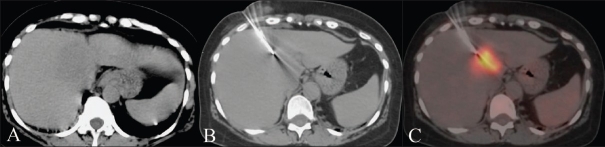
Noncontrast CT scan of the liver (A) during a percutaneous biopsy showing an area of subtle hypodensity which is nearly indistinct from the rest of the liver parenchyma. CT scan (B) shows the needle in place. The accuracy of positioning in the metabolically active lesion is confirmed on the fused PET/CT image (C). The diagnosis was an inflammatory lesion
